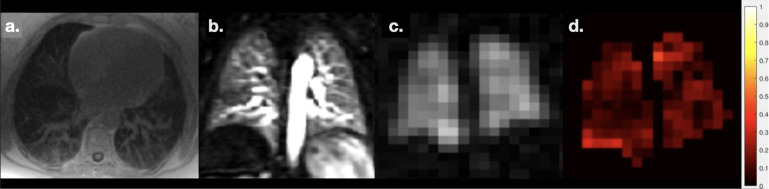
Patient H: Patient in their 60s with difficult ILD. Also diagnosed with fatty liver disease and cirrhosis. CT showed some evidence of unclassifiable ILD and gas transfer was extremely low (TL_CO_ of 26%-predicted). The patient did not meet the diagnostic criteria for hepatopulmonary syndrome and had been assessed for pulmonary hypertension. A ^1^H and HP gas MRI work-up was requested to determine the relative contribution of any ILD or PH or shunting due to hepatopulmonary syndrome, to inform a decision regarding liver transplantation. There was no evidence of shunt on dynamic contrast enhanced MRI angiography. There was mild-to-moderate ILD in the lower lobes with reticular disease seen on UTE (a), normal perfusion on DCE MRI (b) and no substantial ventilation defects on ^129^Xe ventilation imaging (c). The mean RBC/TP (whole-lung average of the map in d.) was low (0.13 compared to 0.47 in healthy volunteers), and RBC/gas was similarly low (0.0017 compared to 0.0036), while TP/gas was high (0.0134 compared to 0.0075), indicating severe interstitial thickening/endothelial diffusion-block and significant gas transfer limitation. MRI assessment supported pulmonary function gas transfer results and confirmed that gas exchange deficiency was the predominant disease process despite discordance with the apparent severity of ILD visible on CT. Key: from left-to-right: a. ^1^H UTE MRI, b. contrast-enhanced perfusion, c. Low resolution ^129^Xe ventilation imaging (obtained during a gas exchange imaging acquisition), d. Map of RBC/TP signal intensities, a metric of gas exchange. ILD, interstitial lung disease; RBC, red blood cell; TP, tissue and blood plasma; UTE, ultrashort time.
